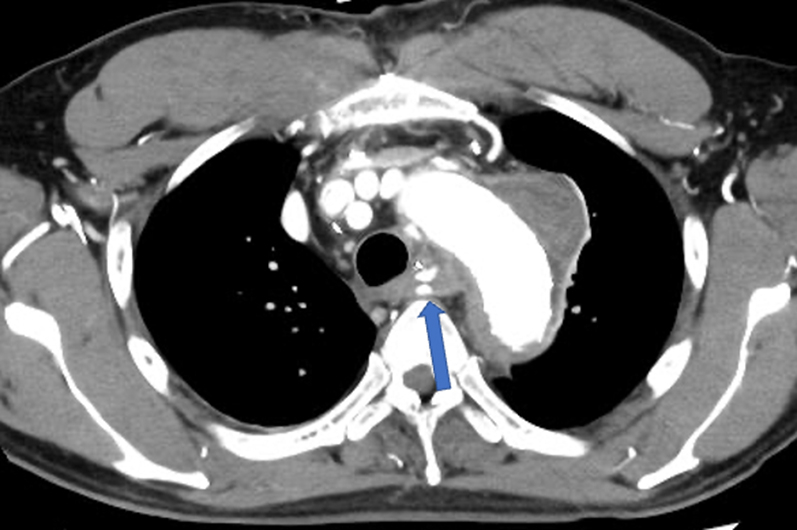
Postoperative computed tomography scan showed aneurysm thrombosis and ligation of the aberrant right subclavian artery reinforced with felt pledgets (*arrow*).
